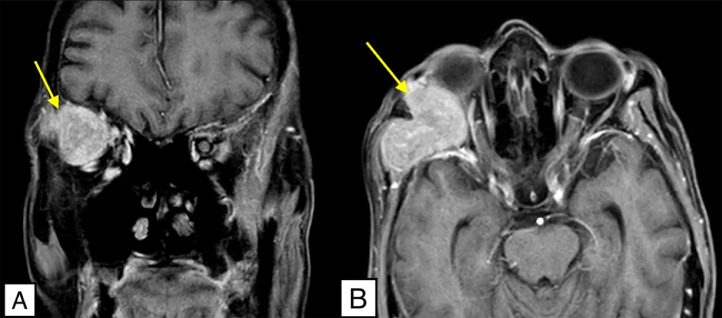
Contrast enhanced magnetic resonance imaging (MRI) of the orbit. (A) Coronal and (B) Axial T1 post-contrast images showing a hyperintense lesion with soft tissue component measuring 3.5 × 3 × 3.5 cm located in the right orbit (yellow arrow). It extends into intraconal region involving right temporalis muscle with lytic destruction of right greater wing of sphenoid and orbital process of frontal bone.
